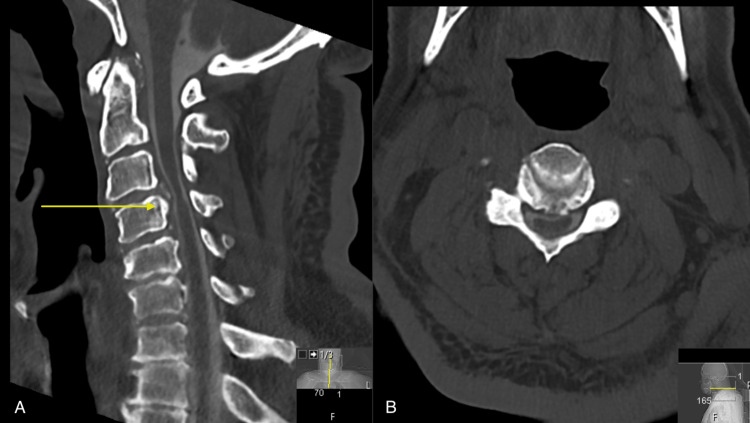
CT Cervical Spine with Myelographic Contrast (A) Single sagittal slice of a CT of the cervical spine with myelographic contrast demonstrating severe spinal canal stenosis secondary to a large disc osteophyte complex which indents the ventral thecal sac and results in compression of the ventral aspect of the spinal cord, at the level of C3-C4 (yellow arrow).  Note: intrathecal contrast is visualized along the ventral and dorsal aspects of the cord, without complete effacement of the CSF space. Furthermore, there are similar findings at C4-C5. (B) Axial view showing bilateral uncovertebral hypertrophy and facet arthrosis, greater on the right side compared to the left, contributing to severe right and moderate left foraminal narrowing.
